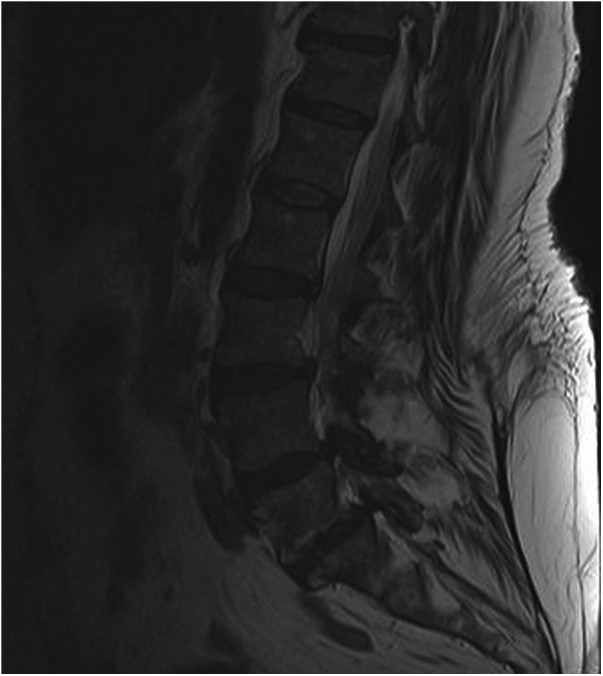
Pre-Operative Sagittal MRI.
